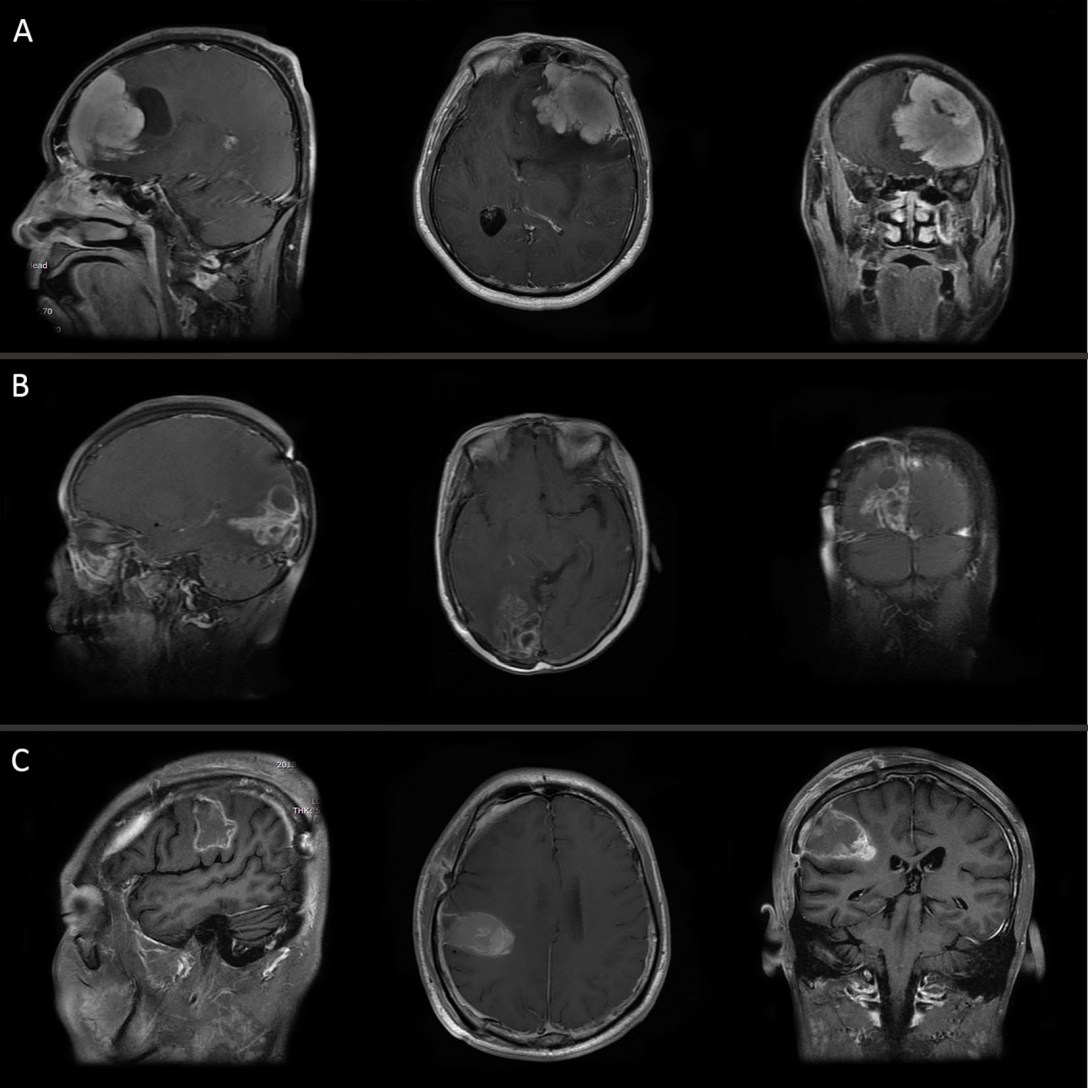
MRI of three pathological subtypes: (A) anaplastic meningioma, (B) papillary meningioma, and (C) rhabdoid meningioma.
